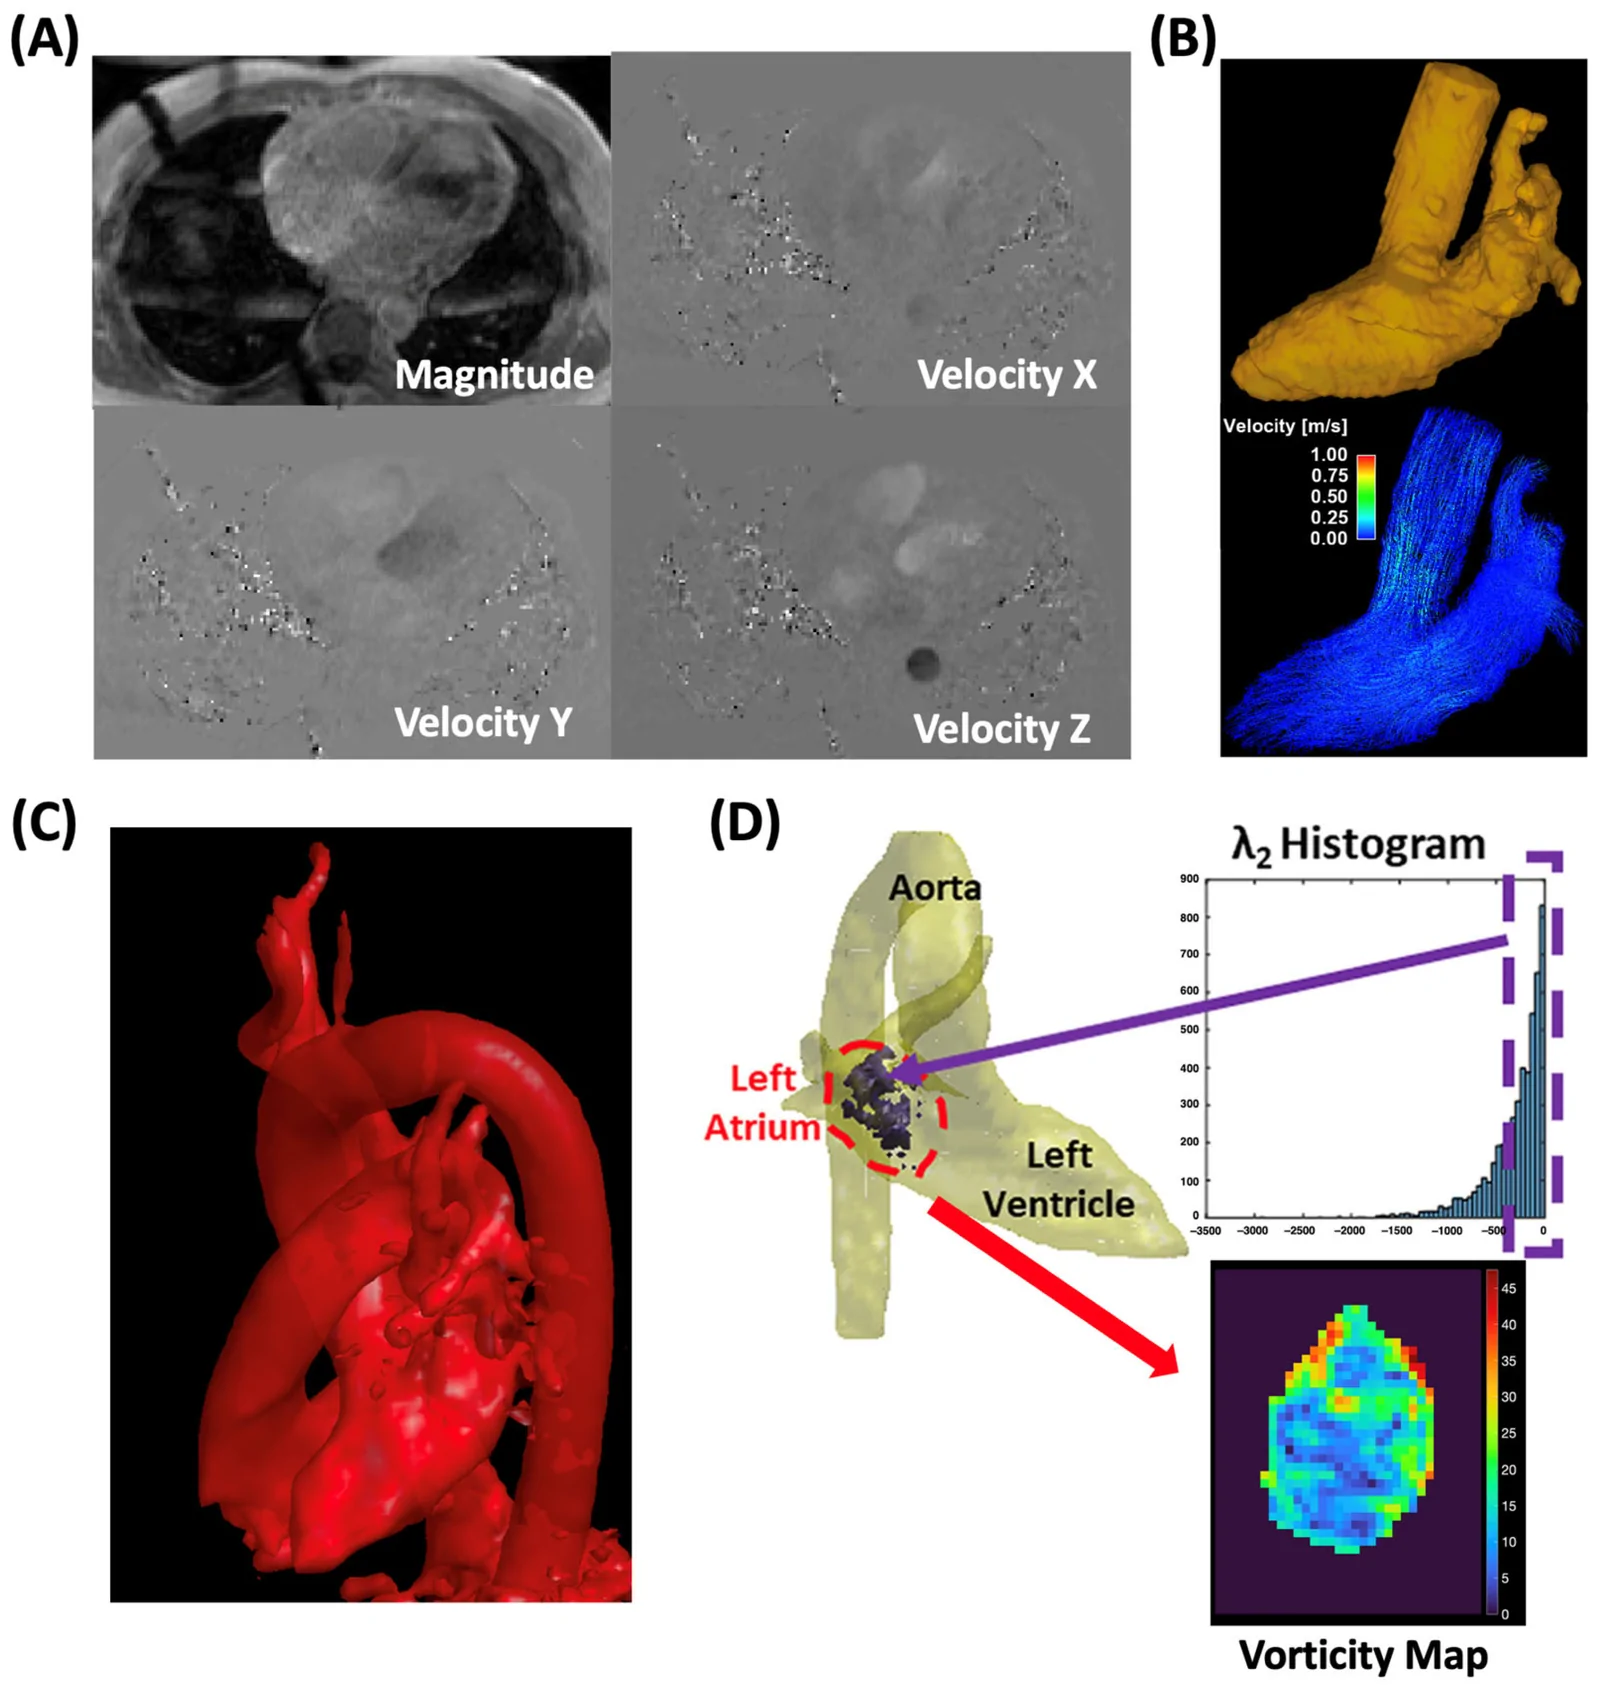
Summary of steps involved in data preparation and image analysis: (A) Sample images derived from 4D flow MRI following correction protocol. (B) Visualization of left heart blood flow dynamics. (C) Time-averaged rendering of phase-contrast magnetic resonance angiography, from which the left atrium (LA) is manually demarcated in successive sections to obtain a 3D segmentation. (D) Vortex volumes derived from LA segmentations using the λ_2_ method, and vorticity values obtained by averaging LA vorticity over the cardiac cycle. Panel D adapted with permission from Ref. [12]. Copyright © 2019, International Society for Magnetic Resonance in Medicine, published by Wiley Periodicals, Inc.
